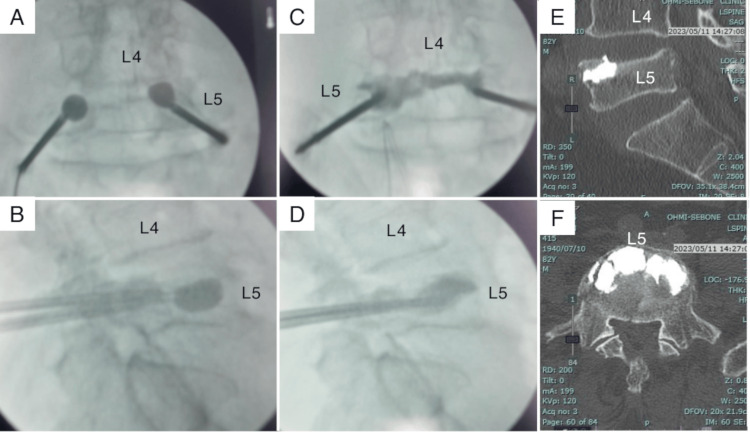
Intraoperative and postoperative images of the initial balloon kyphoplasty (A, B) Balloons were transpedicularly inserted near the fracture. (C, D) A total of 7.3 mL of cement was injected into the vertebral body. (E, F) Postoperative computed tomography images (anteroposterior and lateral projections) confirm cement placement near the fracture.
